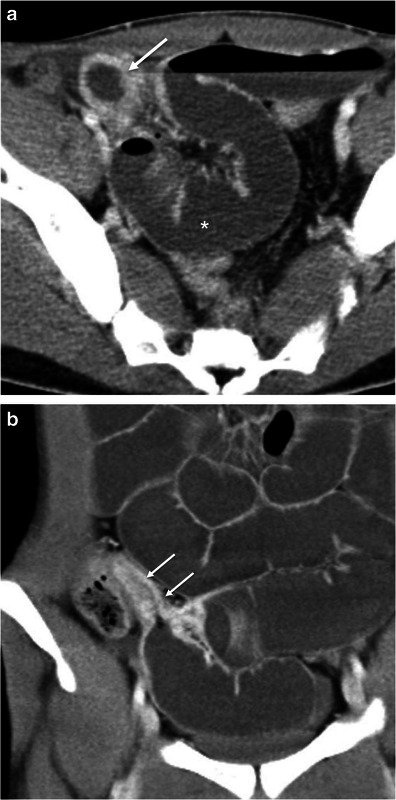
White attenuation pattern in Crohn’s disease. Axial (a) and coronal (b) contrast-enhanced CT scans show homogeneous hyperenhancement (*arrows*) of a thickened and stenotic ileal loop indicating active disease. Note the proximal dilatation of the small bowel loops (*asterisk*) due to the obstruction
